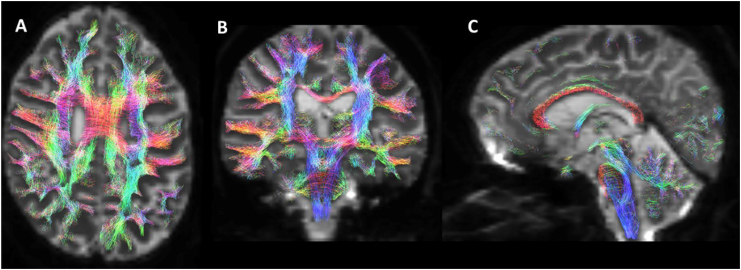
Post-processed diffusion magnetic resonance imaging images (MRtrix3 software) demonstrating association, projection, and commissural fascicles of the central nervous system. Views: A) axial; B) coronal; and C) sagittals. Courtesy of Diogo Fragoso Cardoso.
